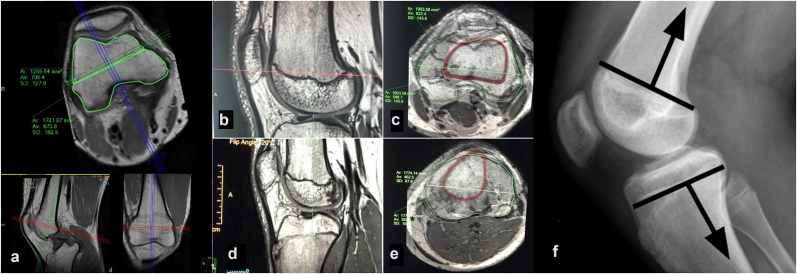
vMRI measurements in a distal femoral physis of a child. We can observe the axial plane at the level of the distal femoral physis of the knee (a). We then calculate the anterior and posterior physeal areas relative to the line that crosses the physis from both medial to lateral tension-band plates holes. In this case, there is a mild uneven distribution of anterior and posterior femoral physeal areas (posterior to anterior area ratio of 1.4). Images b-e show vMRI measurements in a normal, non-intervened knee, so as to find the location of a line that distributes anterior and posterior areas equally. Distal femoral physis in sagittal (b) and axial (c) views. Volumetric magnetic resonance measures in proximal tibial physis in sagittal (d) and axial (e) views. In both axial views we overlap the physeal area (green outline) to the diaphyseal area (red outline); this allows us to translate the optimal position of tension-band plates on true lateral radiographs (e). We suggest that that the line that distributes equally anterior and posterior areas is just anteriorly to the posterior cortex in both femur and tibia.
